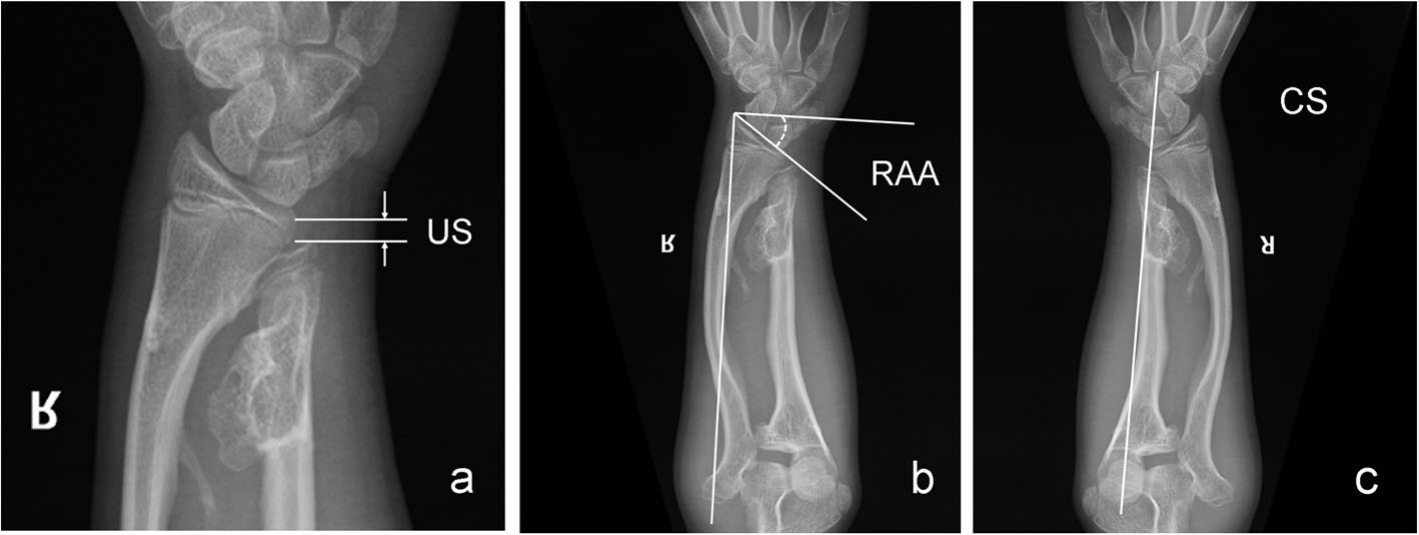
Radiographic evaluation indices proposed by Fogel et al.: a Relative ulnar shortening. b Radial articular angle. c Ulnarward carpal slip. a Relative ulnar shortening (RUS) is measured with the perpendicular line drawn from the distal end of the ulna to the linear axis of the forearm. b The radial articular angle (RAA) is the angle between two constructed lines: one along the articular surface of the radius and the other perpendicular to a line that bisects the head of the radius and passes through the radial edge of the distal radial epiphysis. The normal RAA is 15°-30°. c Ulnarward carpal slip (CS) or displacement of the lunate off the radius is measured as the percentage of contact of the lunate with the radius. An axial line drawn from the center of the olecranon through the ulnar edge of the distal radius normally bisects the lunate. Carpal slip is considered abnormal when the lunate is displaced ulnarward by > 50%
